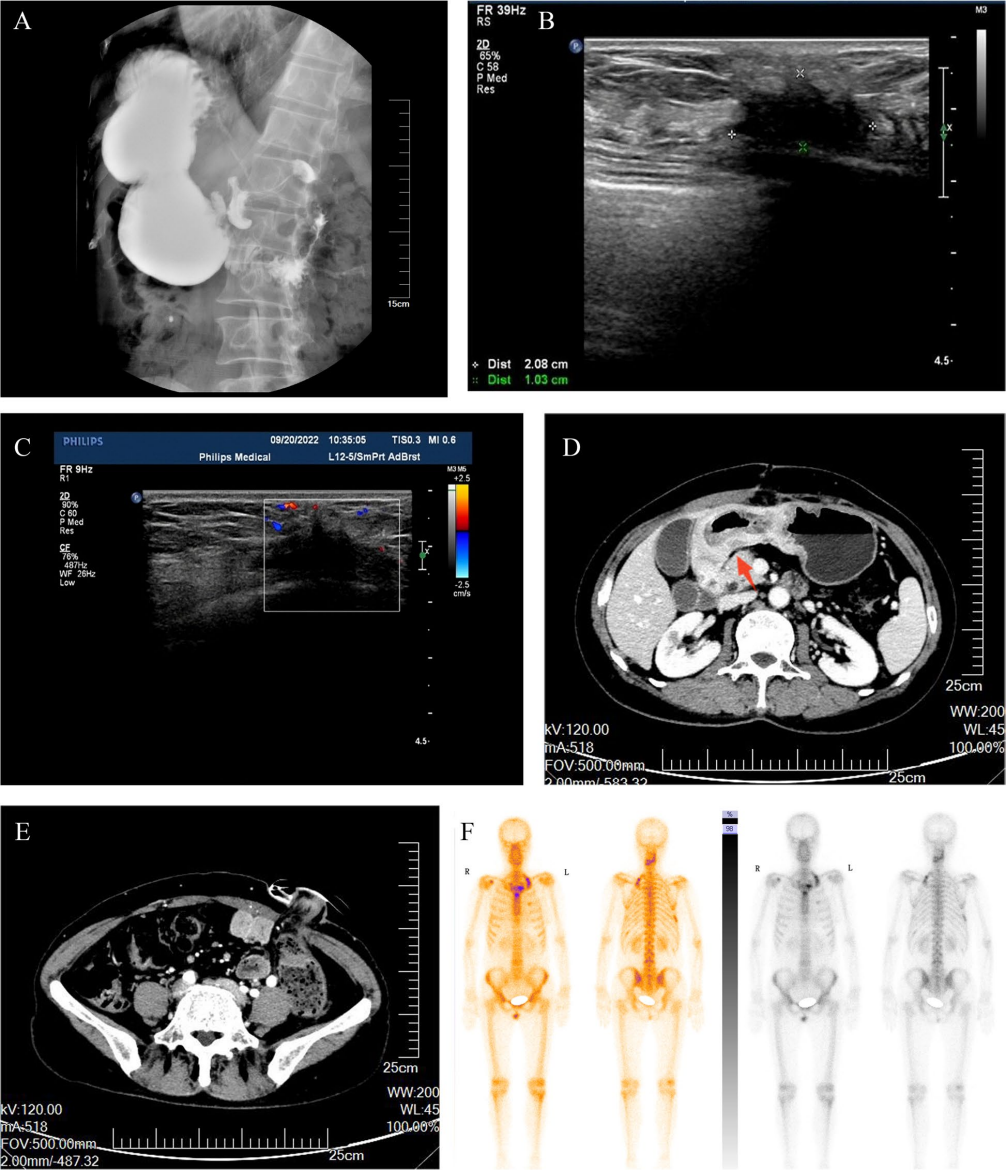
Preoperative upper gastrointestinal X-ray barium meal showed gastric retention, gastroduodenal anastomosis stenosis, and obstructed contrast passage (A). Breast ultrasound showed a hypoechoic cluster of about 2.1 cm*1.0 cm in size, with poorly defined and irregular shape, and dotted strong echogenicity in the right breast next to the nipple at point 7, and CDFI: blood flow signal was visible (B, C). Postoperative gastric CT showed thickening of the gastric wall in the sinus, the thickest part of which was about 22 mm in diameter, involving the whole circumference of the lumen, with a length of about 150 mm, and the surrounding lymph nodes showed that the obstructive symptoms were significantly relieved after surgery (D, E). The whole-body bone scan suggested multiple tumor metastases (F)
